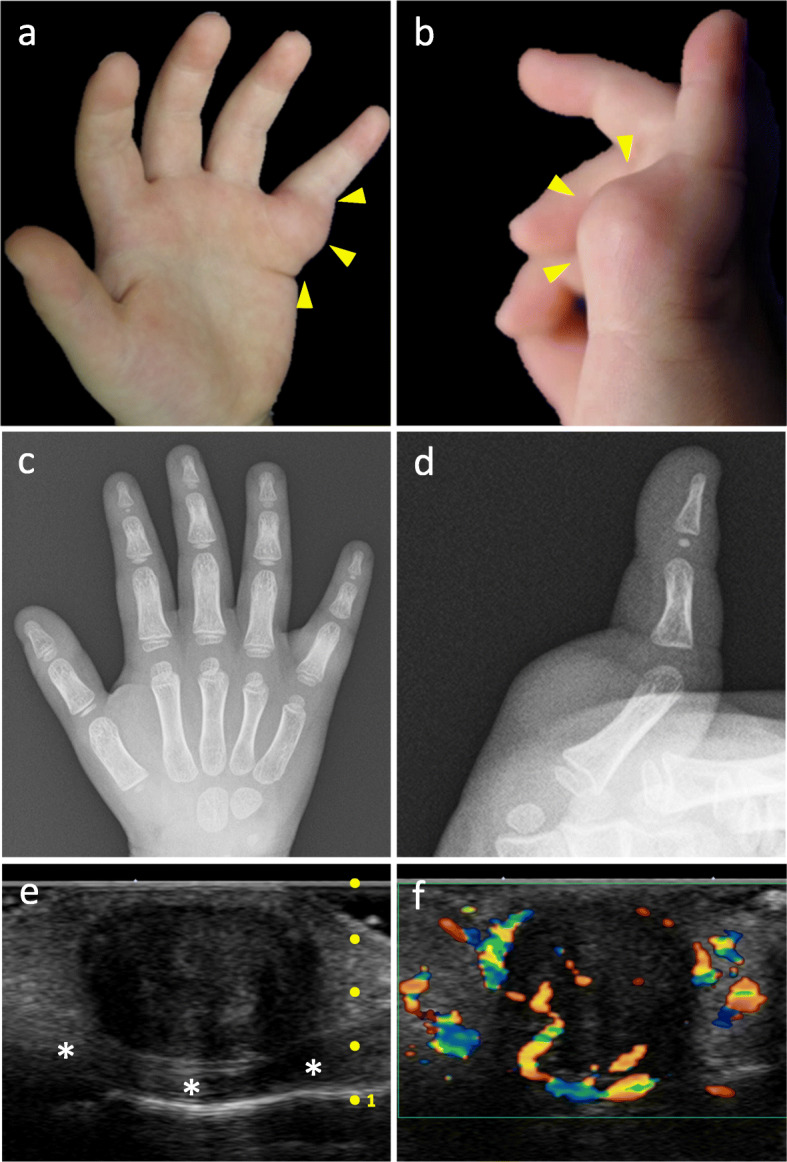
a, b The mass (yellow arrowheads) is located on the volar aspect of the left little finger from the distal palmer crease to the proximal interphalangeal crease. c, d Anteroposterior and lateral radiographs show a circumferential soft tissue shadow on the proximal phalange of the left little finger. Bony abnormalities are not observed. e Ultrasonography demonstrates a well-circumscribed heterogeneous low echoic lesion. The lesion is located on the flexor tendon (white asterisks) and the boundary is clearly maintained. f Color Doppler ultrasonography shows blood flow into the lesion
